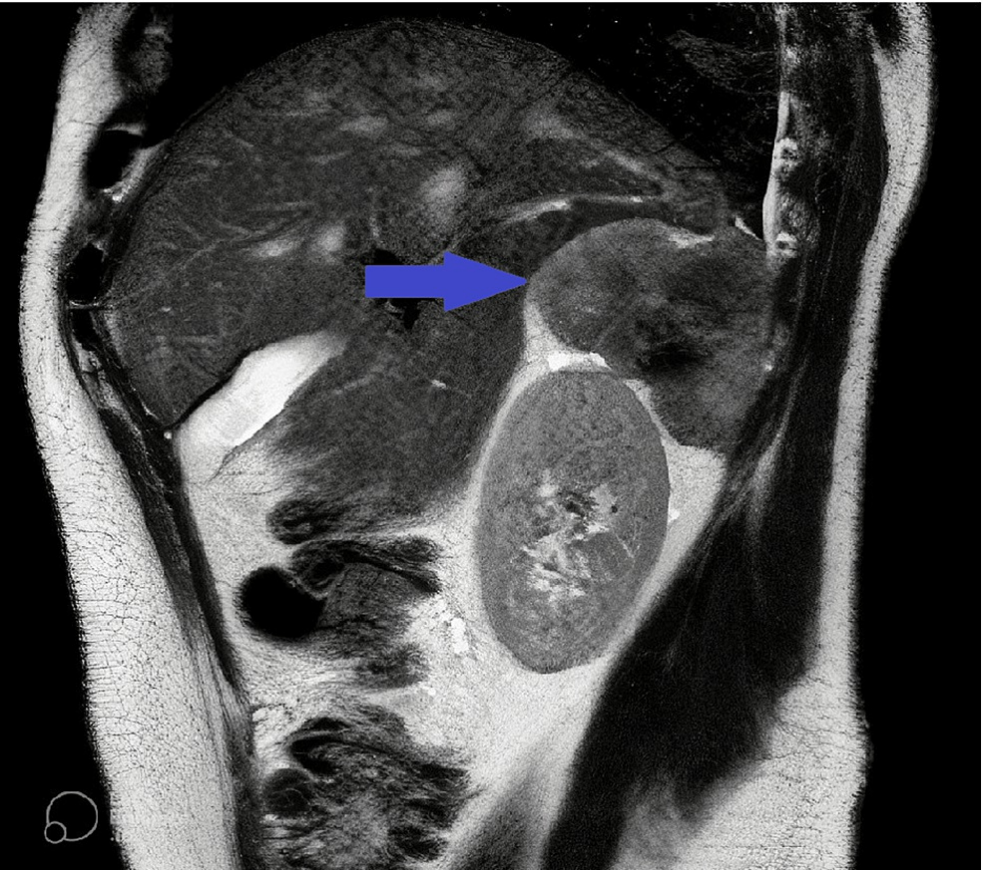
Magnetic resonance image of the retroperitoneal synovial sarcoma localized between the liver, diaphragm, and upper pole of the right kidney.
